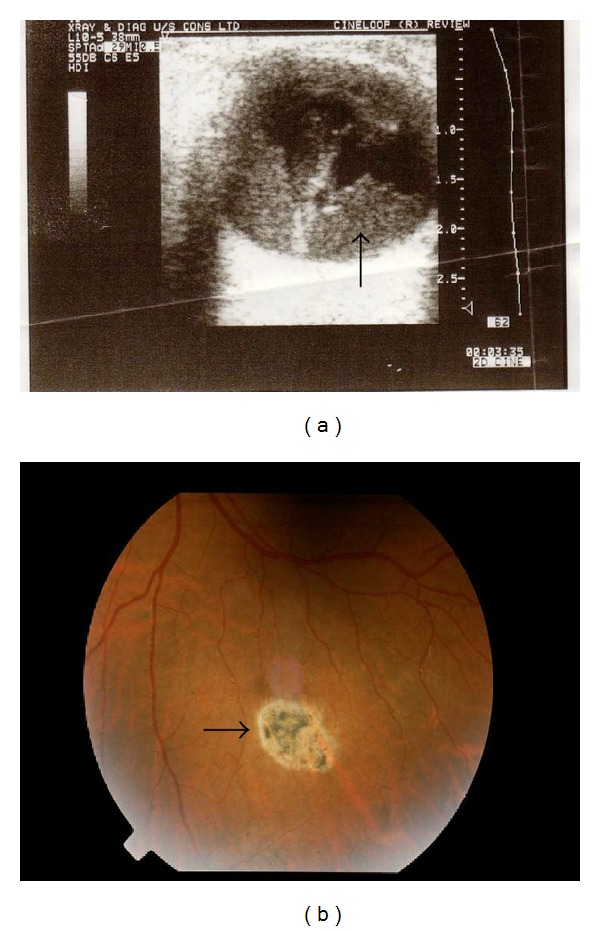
(a) Ultrasound image of the left eye of the patient demonstrating vitreous hemorrhage. (b) Black starburst in peripheral retina of left eye.
